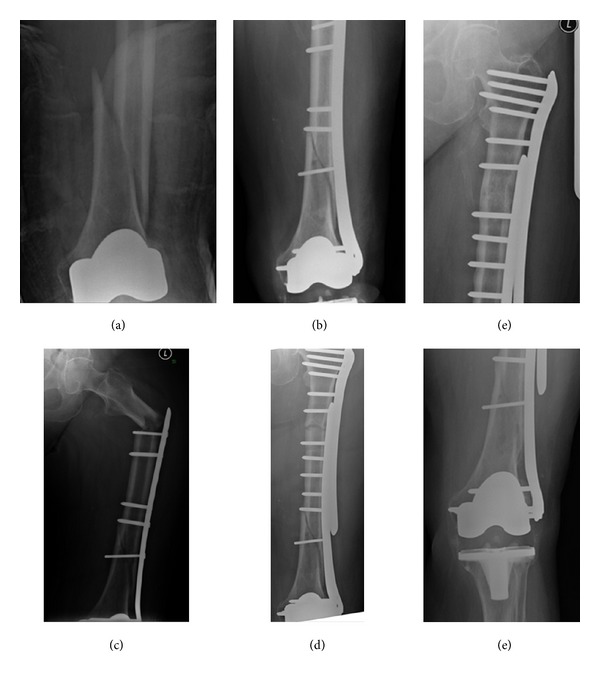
Case 1: (a) Periprosthetic fracture femur, (b) distal femoral plate (DFP) for the treatment of 1st fracture, (c) fracture proximal to the DFP plate, (d) postoperative X-ray, (e) healing of both fractures-DFP over DFP.
